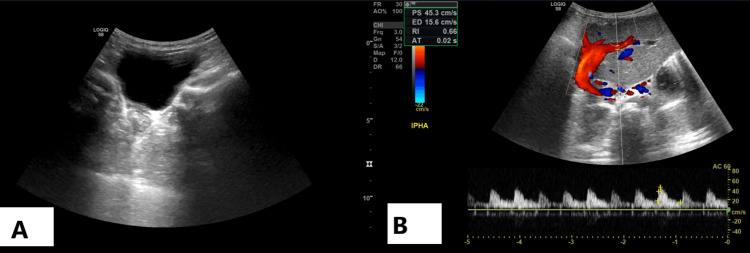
Doppler ultrasound of transplanted liver The Doppler images show normal antegrade flow in the hepatic artery, hepatic vein, and portal vein; (A) shows a normal fluid-filled urinary bladder, and (B) shows patent flow through liver vasculature.
